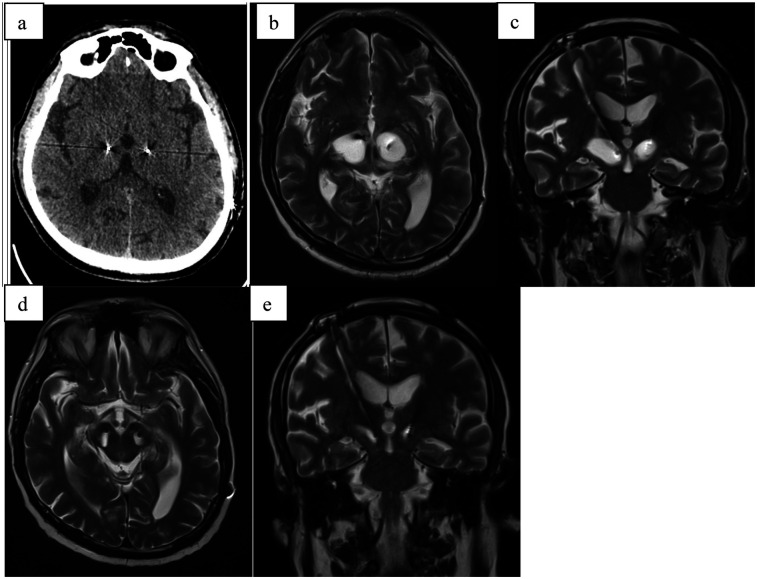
(a) CT scan directly after electrode implantation; (b) MRI-T2 transversal scan 2 months after stimulation initialization (3 months after surgery) with hyperintensive cystic formation surrounding both electrodes; (c) MRI-T2 coronal scan 2 months after stimulation initialization (3 months after surgery) with hyperintensive cystic formation surrounding both electrodes; (d) MRI-T2 transversal scan 4 months after stimulation initialization (5 months after surgery) with smaller hyperintensive cystic formation surrounding both electrodes after steroid treatment; (e) MRI-T2 coronal scan 4 months after stimulation initialization (5 months after surgery) with smaller hyperintensive cystic formation surrounding both electrodes after steroid treatment.
